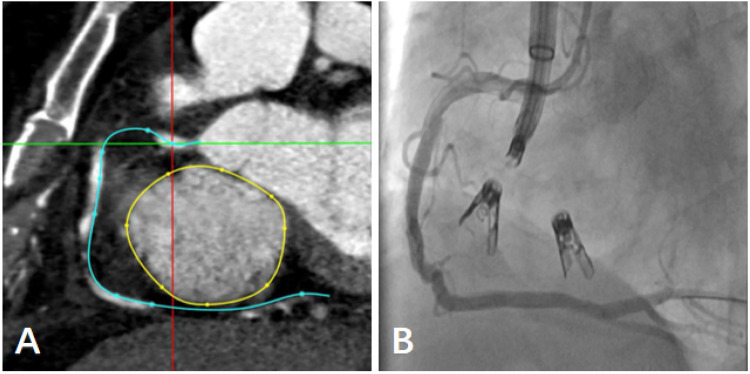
(A) The coronary CTA: the distance between the RCA and the tricuspid annulus is all greater than 3.0 mm. (B) After the tricuspid annuloplasty re-examination of right coronary angiography: TIMI grade 3 blood flow.
